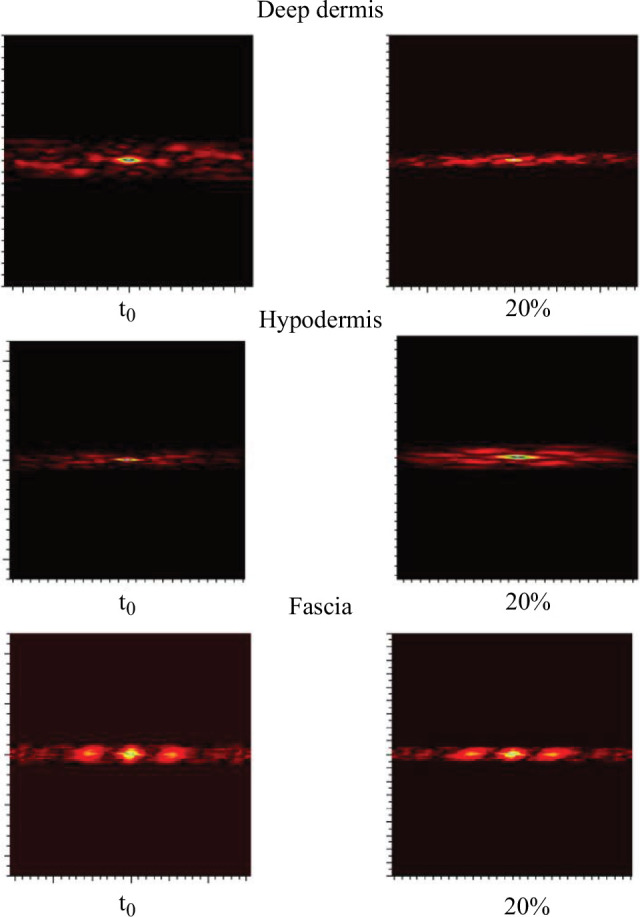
Autocorrelation images of the ultrasound scan images for each layer, deep dermis, hypodermis and fascia. On the forearm (*n* = 1 and man older than 30 years) following the same approach as for the relaxation tests (see protocol given in §2.2).
